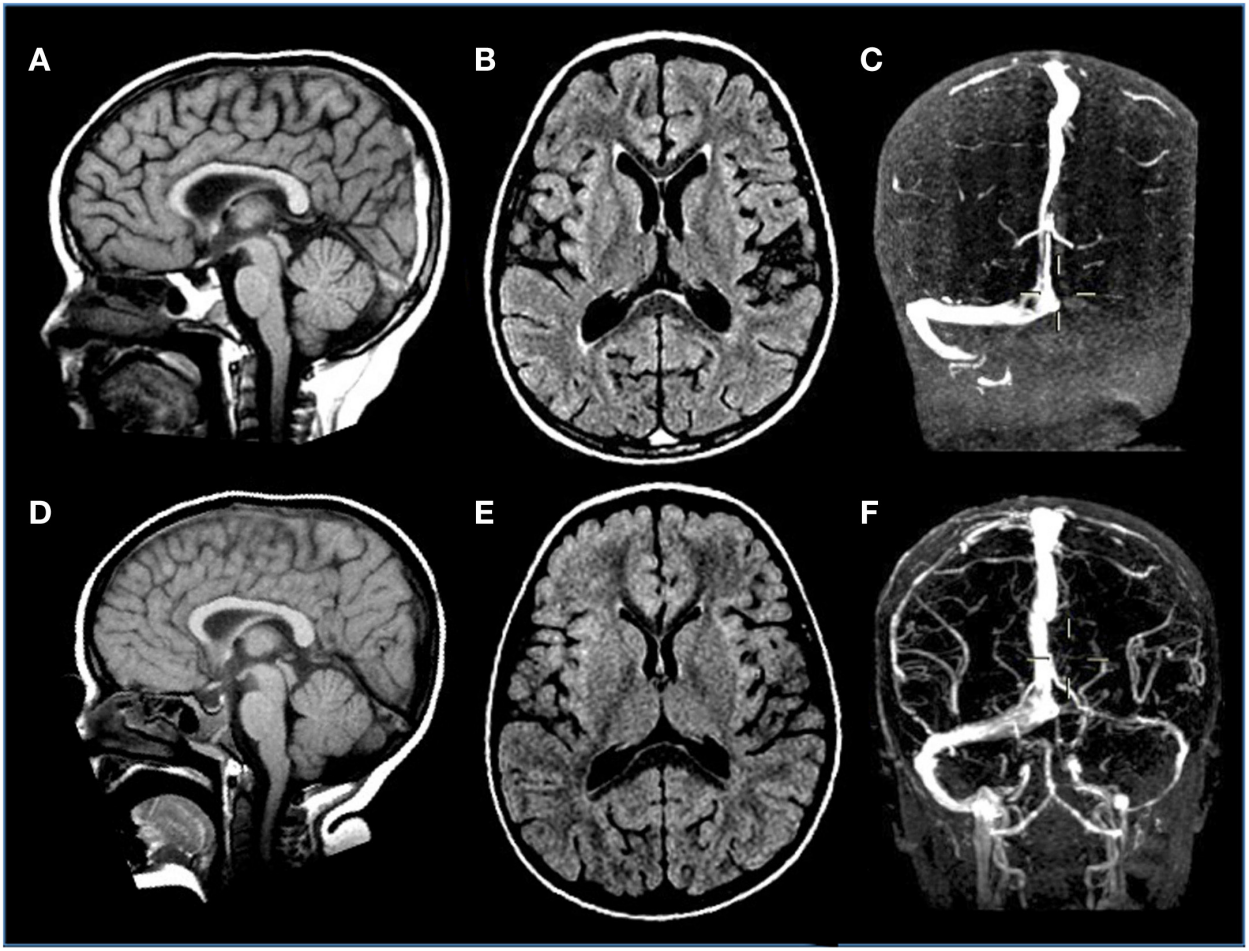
Venous sinus thrombosis in a 4-year-old boy with acute lymphoblastic leukemia treated with l-asparaginase. (A–C) Sagittal T1, axial FLAIR T2-weighted, and coronal angio-MR images display thrombosis of the distal portion of the superior sagittal and left transverse sinuses. (D–F) Sagittal T1, axial FLAIR T2-weighted, and coronal angio-MR images acquired 3 weeks later reveal resolution of the thrombosis with almost complete restoration of flow signal within the previously thrombosed sinuses.
